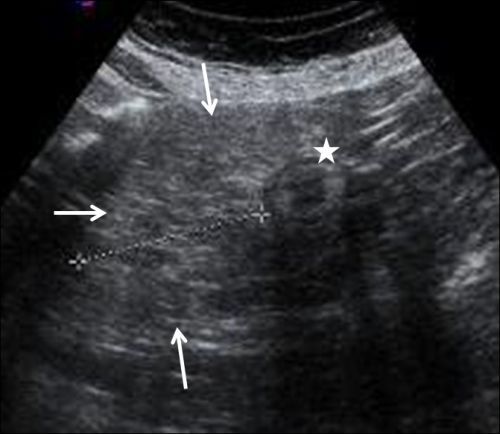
Pelvic ultrasound examination showing hyperechoic fat tissue (white arrows) surrounding the sigmoid colon (star).
